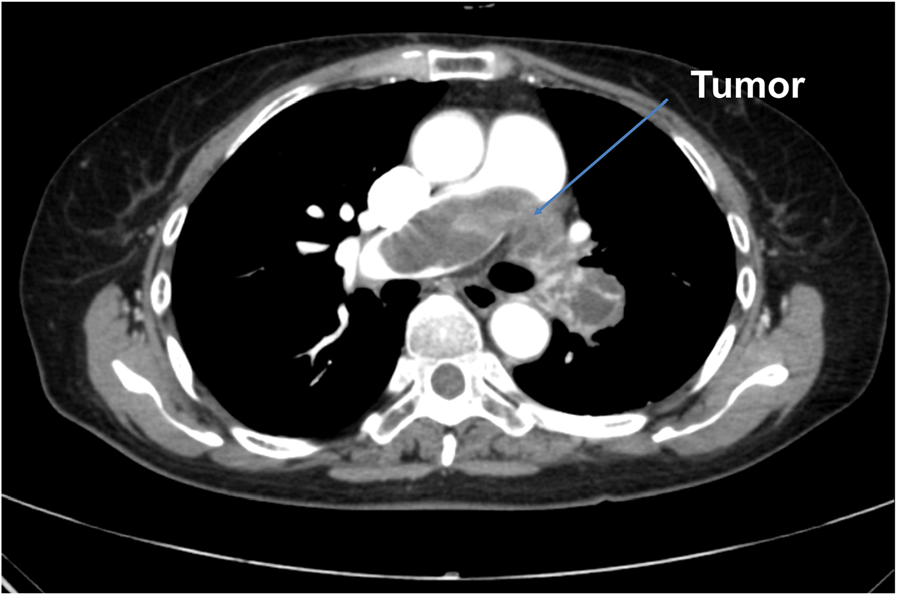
A tumor in the pulmonary artery was observed on chest computed tomography imaging. The mass filled the left pulmonary artery, from its distal portion and extended to the right pulmonary artery, which had minimal blood flow
